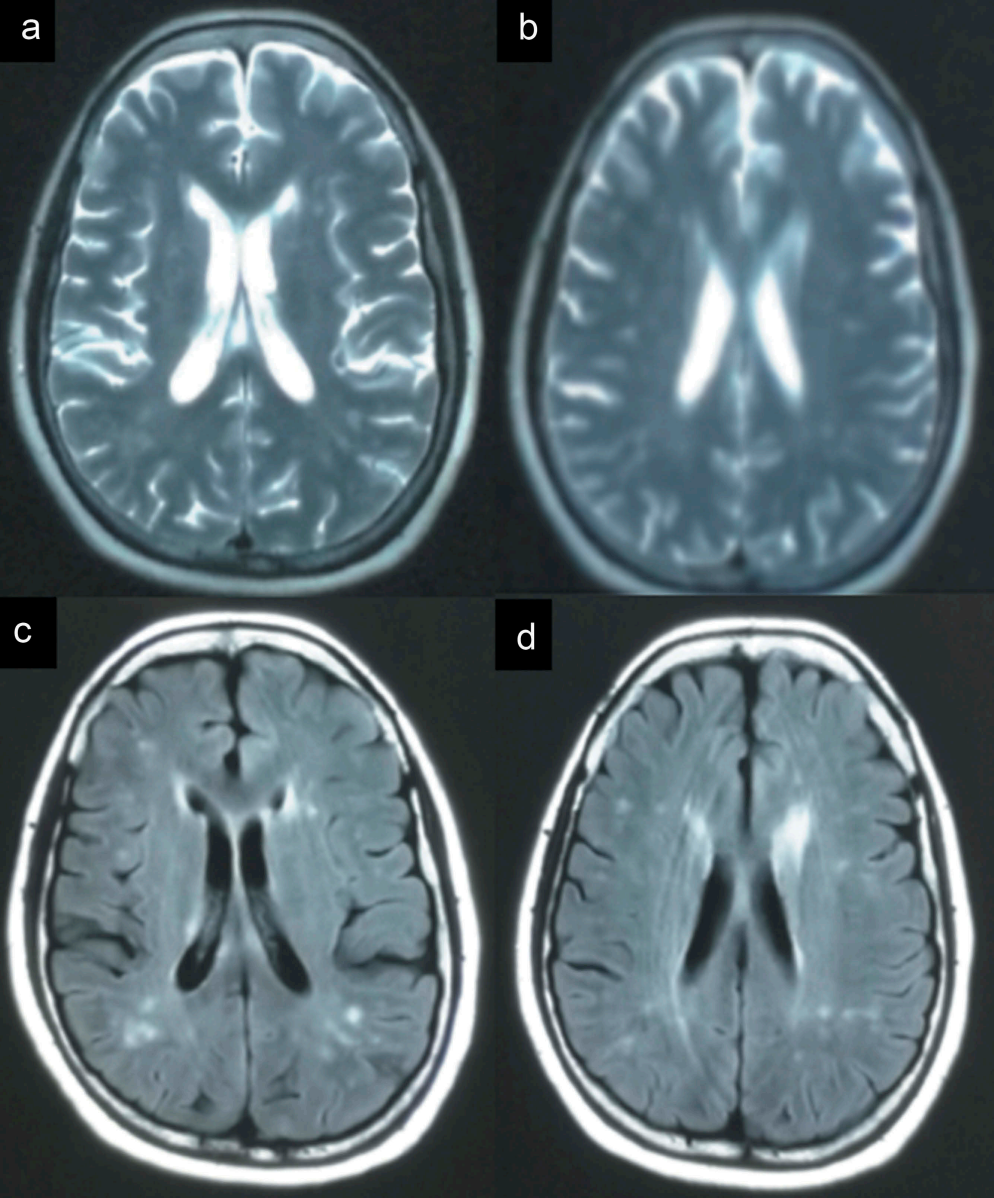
The imaging of the patient. MRI showed abnormal signals in the bilateral frontal subcortical areas and the periventricular area of the lateral ventricles.
